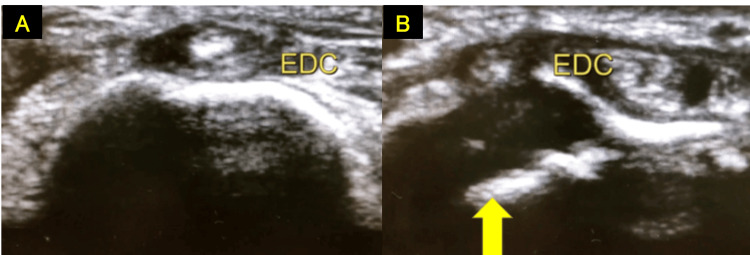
Ultrasound of the wrist showing EDC entrapment within the medullary cavity of the radius A: Normal. B: EDC entrapment within the medullary cavity of the radius (yellow arrow). EDC, extensor digitorum communis
